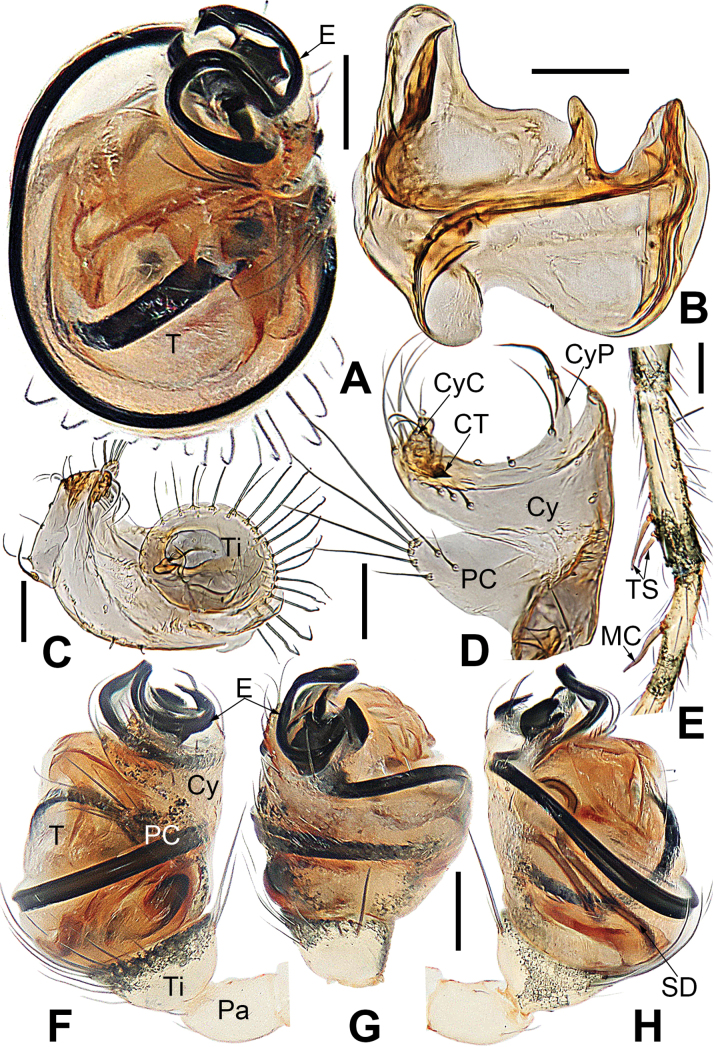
Microdipoenazhulin* sp. nov. from Guangxi of China A palp, apical B conductor, dorsal C cymbium, apical D cymbium, prolateral E left tibia I and metatarsus I, prolateral F palp, prolateral G palp, ventral H palp, retrolateral. Abbreviations: CT = cymbial tooth, Cy = cymbium, CyC = cymbial conductor, CyP = cymbial process, E = embolus, MC = mating clasper, Pa = patella, PC = paracymbium, T = tegulum, Ti = tibia, TS = tibial spine. Scale bars: 0.10 mm.
